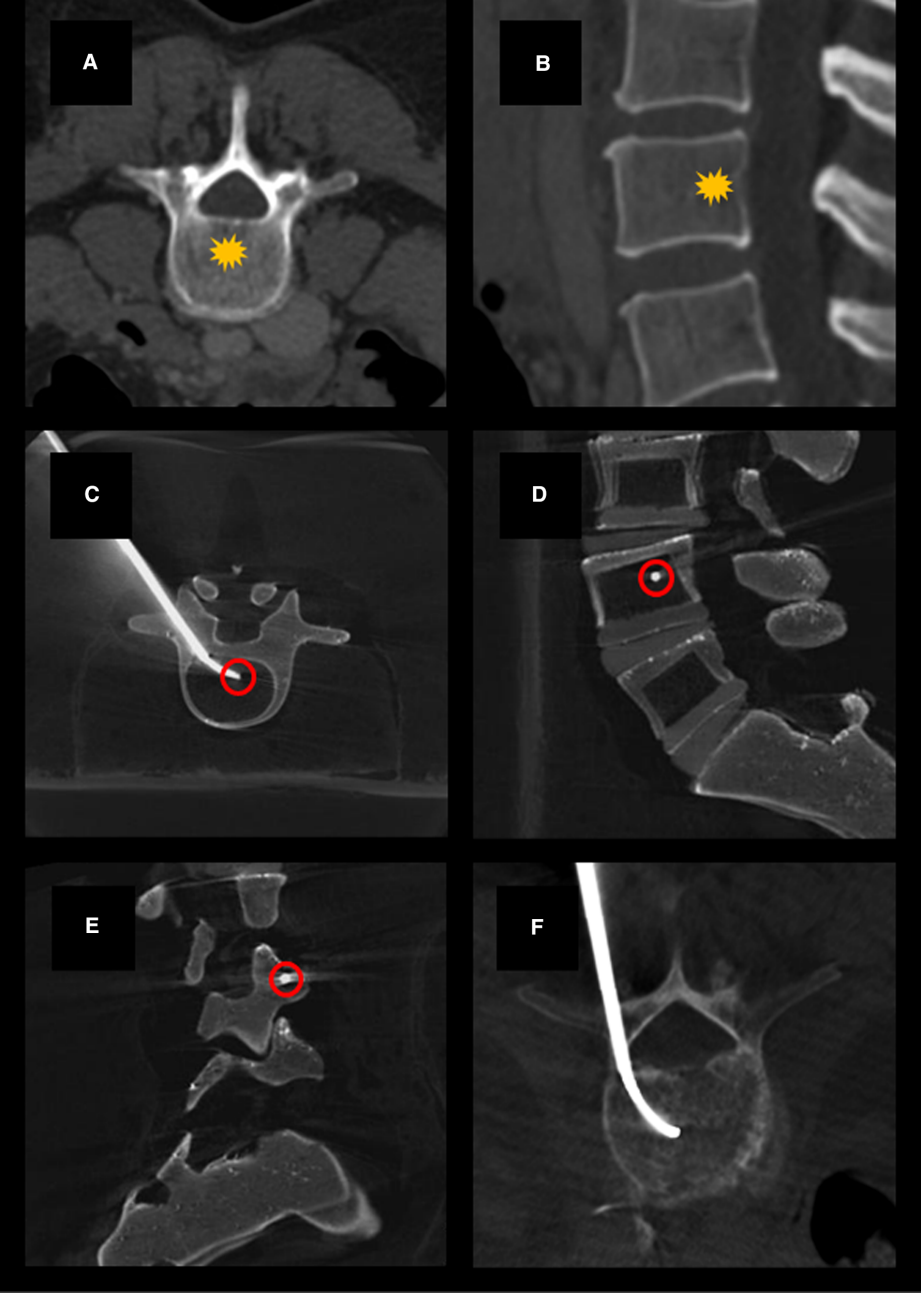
Example axial (A) and sagittal (B) CT images demonstrating normal vertebral bodies and the expected location of the basivertebral nerve (yellow star). Example cone-beam CT images acquired using a Medtronic Kyphon® training model showing transpedicular curved canula placement on axial (C), lateral (D), and oblique lateral (E) views. Canula tip placement (red circles) is ideally centrally located within the vertebral body on AP imaging and between 30% and 50% of the anterior-to-posterior length of the vertebral body from the posterior wall on lateral view. Example showing curved canula placement in a patient undergoing kyphoplasty with BVN block (F).
